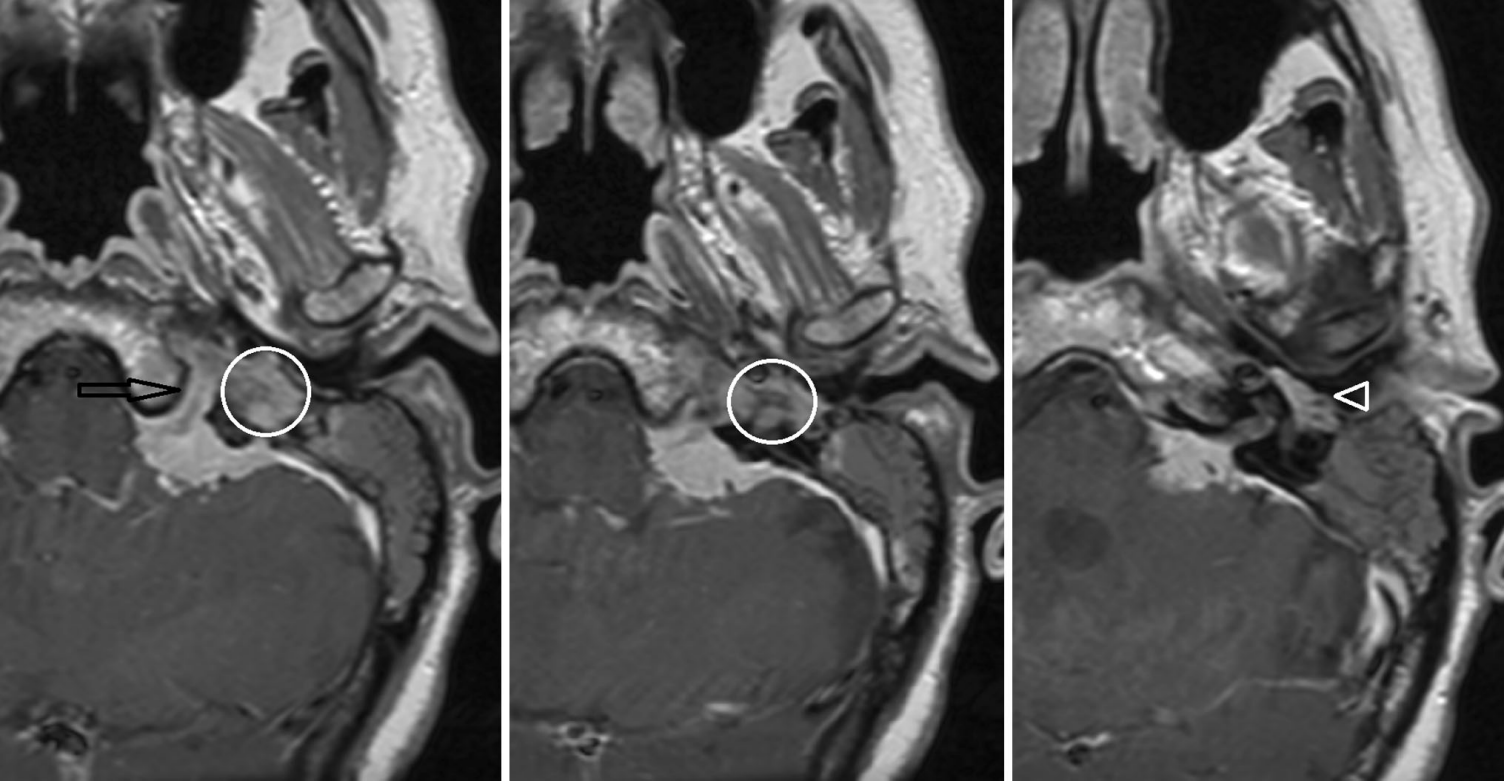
Meningeoma on MRI. Axial contrast-enhanced T1-W images. Enhancing mass located in the left cerebellar-pontine angle with extension into hypoglossal canal (*arrow*), jugular plate (*encircled*), and the middle ear (*arrowhead*)
